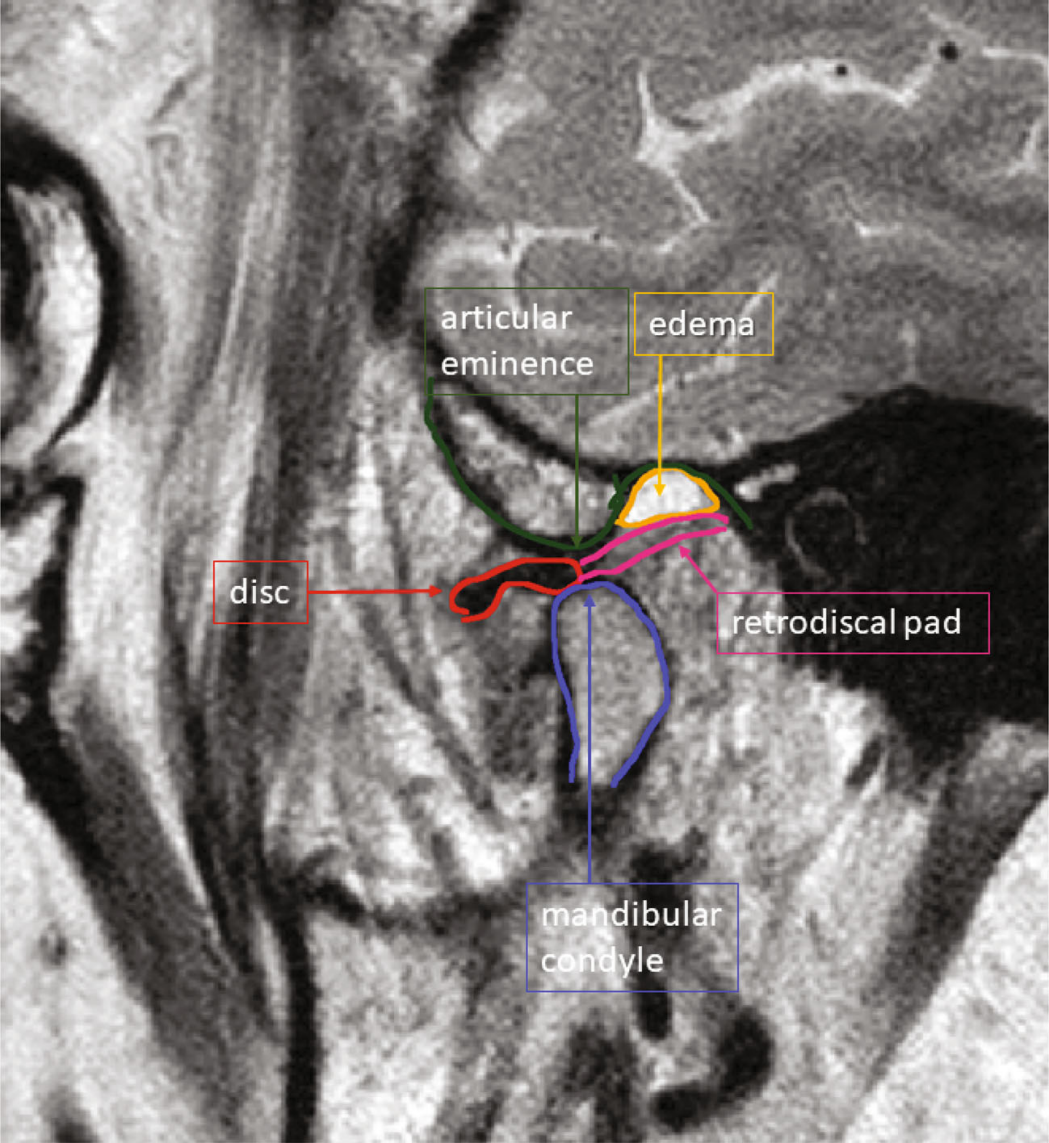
Initial MRI of the left TMJ with the patient's mouth open revealed anterior disk displacement of the left articular disk with significant anatomical deformity (thickening of the posterior edge) and effusion in the left TMJ. The dynamic sequences revealed limited condyle movement, disk deformity, and displacement without reduction on opening. Joint effusion was present.
